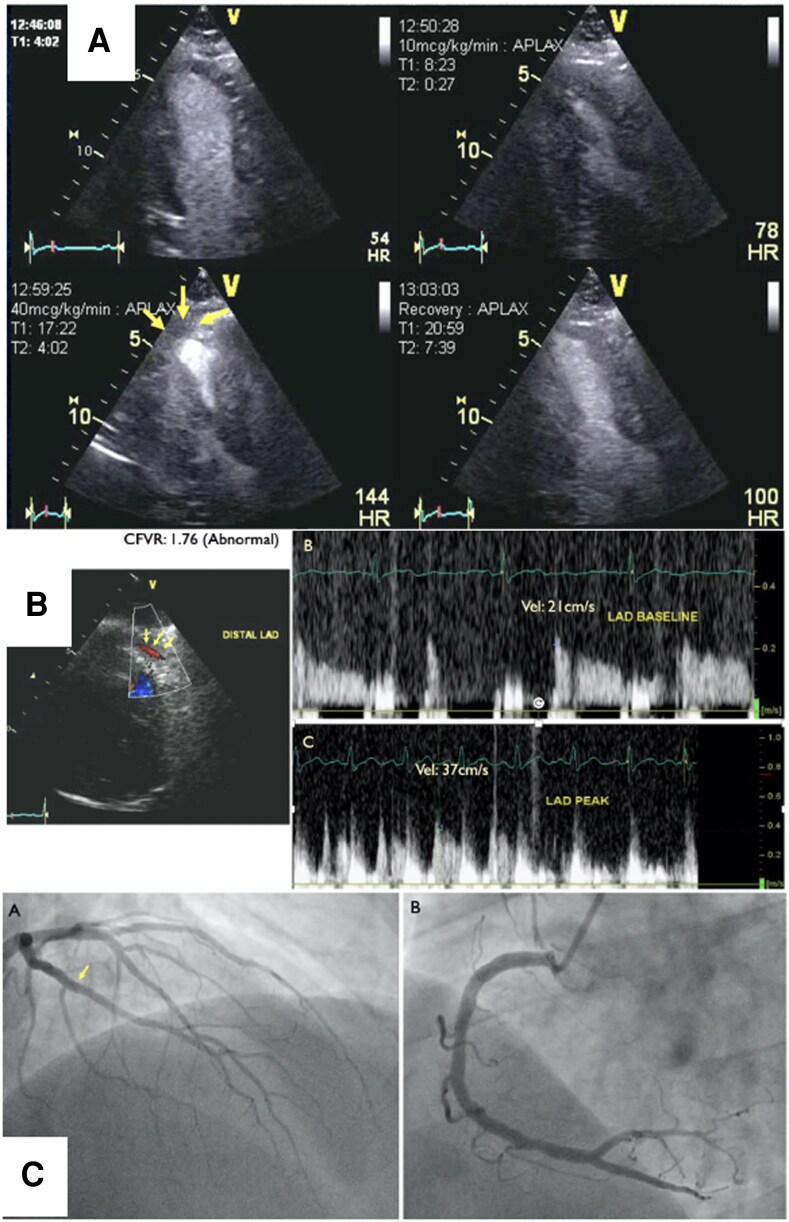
Case of a 53-year-old man with history of angina and prior PCI—for recurrence of typical angina. (*A*) Dobutamine Stress Echocardiography: Test was terminated at the end of protocol at 86% of target heart rate due to progressive angina. No resting RWMA, and all segments of the left ventricle augmented well during low dose. Peak stress: the apical segment became thin and dyskinetic (arrows). (*B*) The CFV in the distal LAD was 21 cm/s and 37 cm/s at baseline and peak, in keeping with reduced CFVR: 1.76. (*C*) Invasive coronary angiography confirmed non-occlusive CAD—diagnosis of ANOCA was made.
